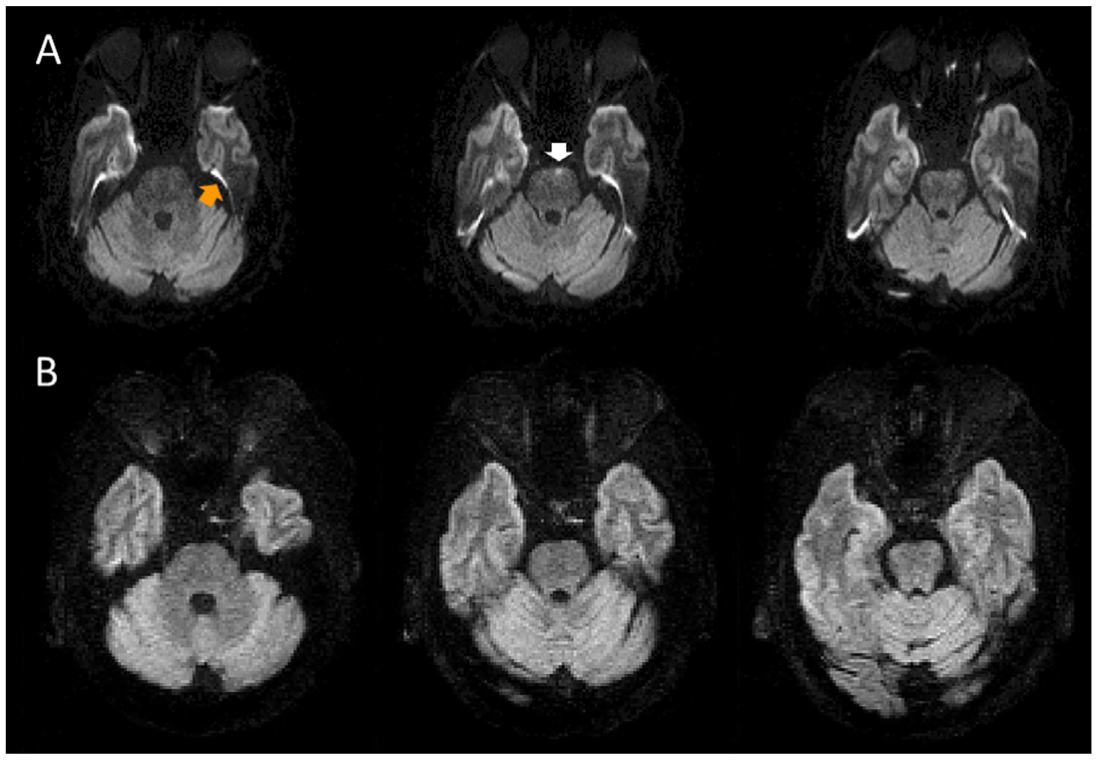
Artifacts on the DWI sequences. A) Example of artifacts on the high-resolution EPI-DWI (three consecutive slices shown). A typical susceptibility artifact is visible on the EPI-DWI (orange arrow)–note that such artifacts were ignored by the raters and are not the focus of this study. An intraparenchymal hyperintensity in the left pons (white arrow) is seen on a single slice. B) Neither of these artifacts is present on the STEAM-DWI.
